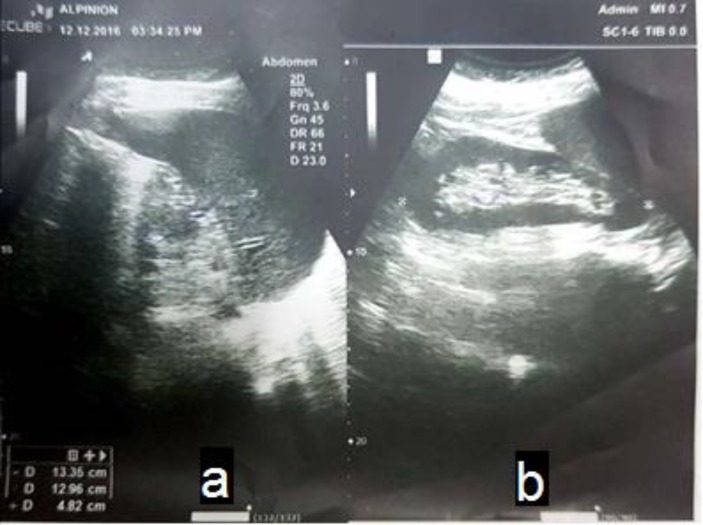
a) The abdomen sonography shows increased echo in spleen parenchyma. b) The abdomen sonography shows the spleen size enlargement
